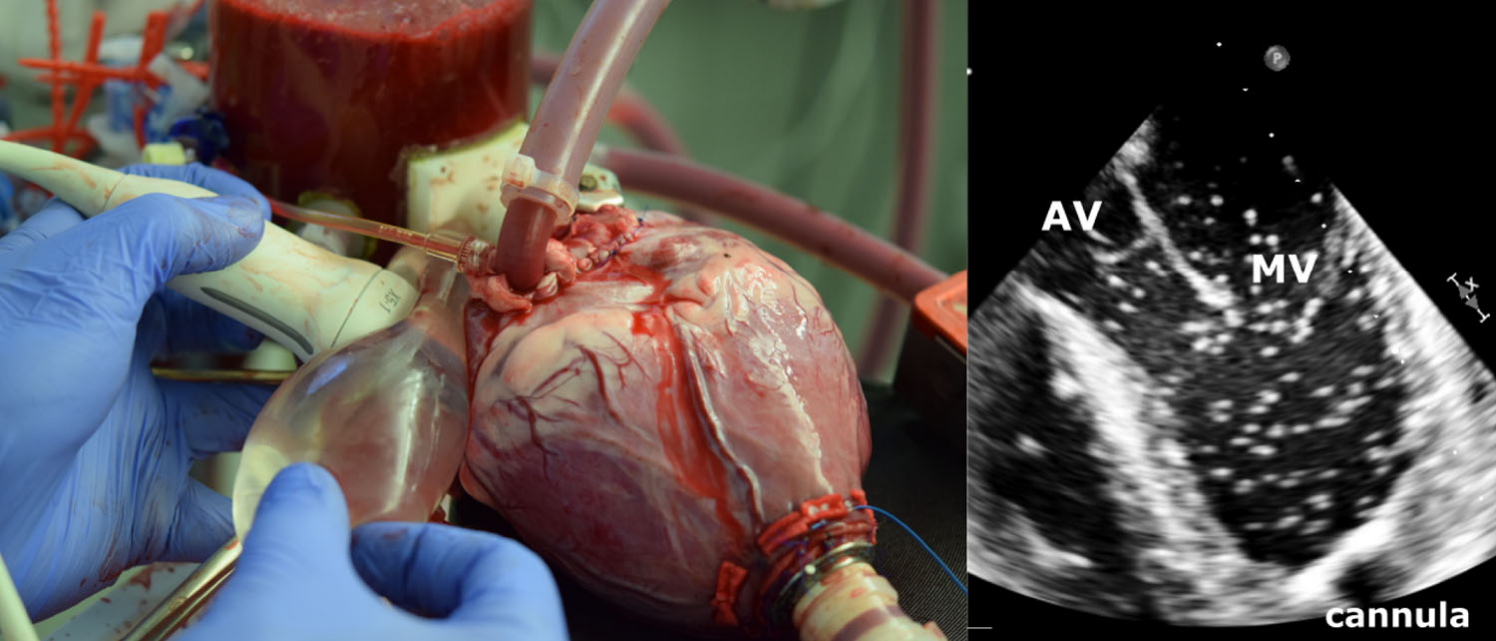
Left: use of the fluid-filled silicone pouch for improved contact with the cardiac surface and compensation of cardiac motion. Right: exemplary echocardiography image of the left ventricle during application of the microbubbles.
